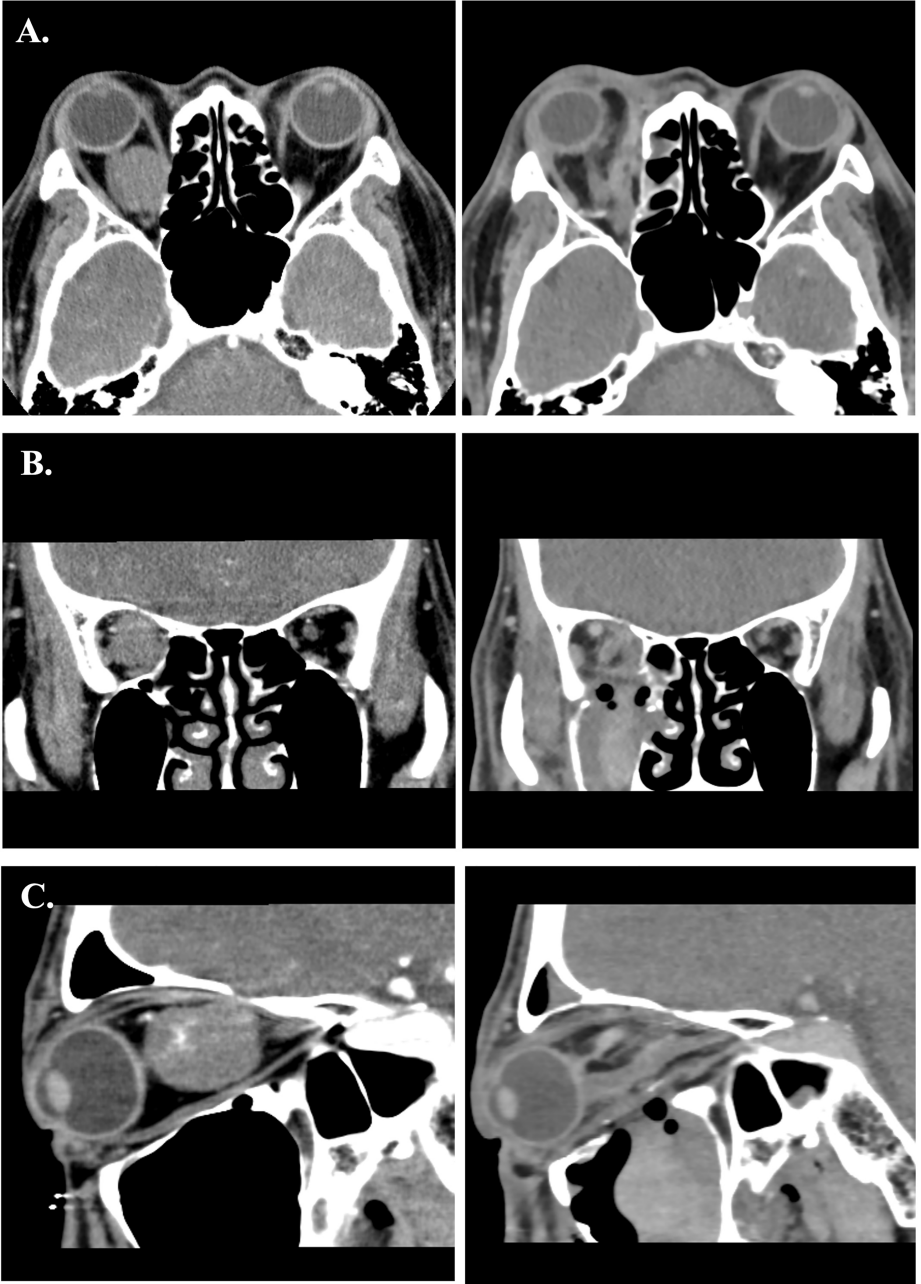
(A–C) Preoperative and postoperative images of Case 4. The left side axial, coronal and sagittal images were the preoperative CT scans. The right-side immediate postoperative CT images showed swelling of the medial rectus and mild distortion of medial rectus muscle.
